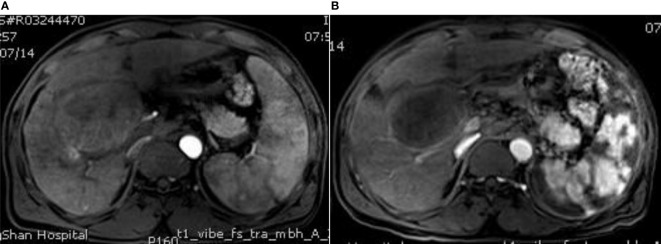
MRI image before surgery and MRI image after surgery. (A) The contrast-enhanced MRI of the liver shows multiple space-occupying lesions, the larger lesions are located in the right lobe of the liver, which adjacent to the hepatic hilum, moderate enhancement and splenic enlargement can be observed in the arterial phase; (B) Enhanced MRI of the upper abdomen at 3 months after surgery showes that the liver tumor shrank without obvious enhancement, the spleen shows piebald necrosis, the spleen infarct area accounts for about 50%, and the platelets and leukocytes increase to the normal level and are stable.
